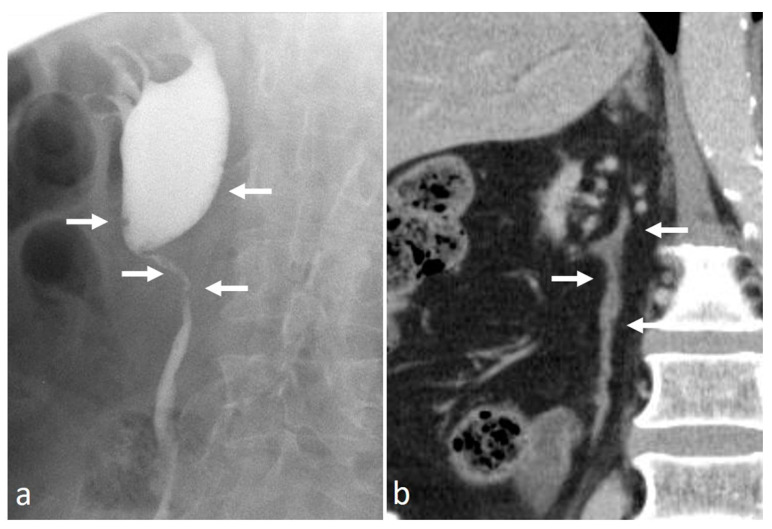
Pyeloureteritis cystica: ascending pyelography (a) and CT nephrographic coronal CT (b) images. Multiple small and regular filling defects are recognizable in the renal pelvis and proximal ureter (arrows in (a)), which correspond to tiny hypodense lesions inside a diffuse enhancing urothelial wall thickening (arrows in (b)).
